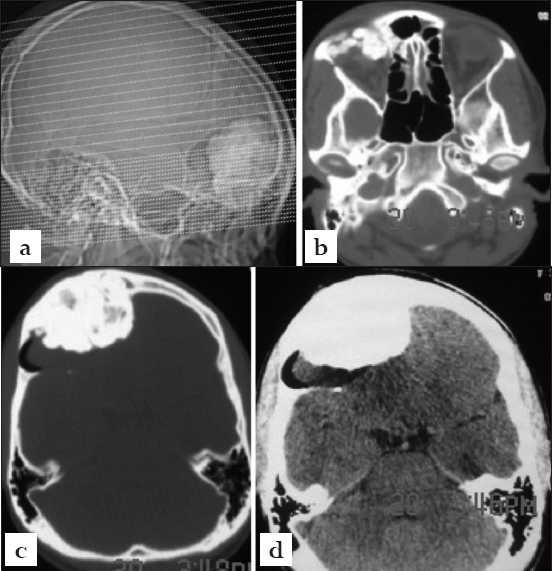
Preoperative imaging, cranial CT scanning. (a) CT scanogram showing the huge highly calcified frontal-ethmoidal mass with a cresentic radiolucent mass (mucoceles) capping its posterior rim (b) the mass involved the right orbital superior rim and roof (c) the mass is associated with expansion of the adjoining diploe suggesting the differential of intradiploic dermoid and (d) there is marked compression of the frontal lobes especially on the right
